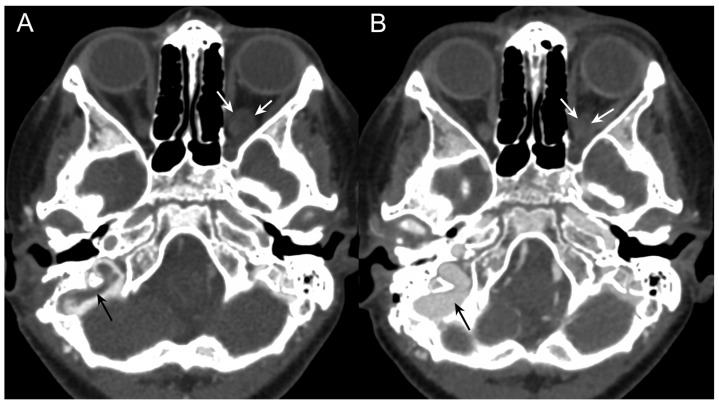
(A): CT obtained a few days after placement of the right transverse sinus stent shows residual thrombus (black arrow) and a reduction in the size of the intraorbital meningocele (white arrows). (B): At follow-up CT acquired after one month, complete patency of sigmoid sinus is achieved (black arrow). A further reduction in the size of the meningocele is shown (white arrows).
